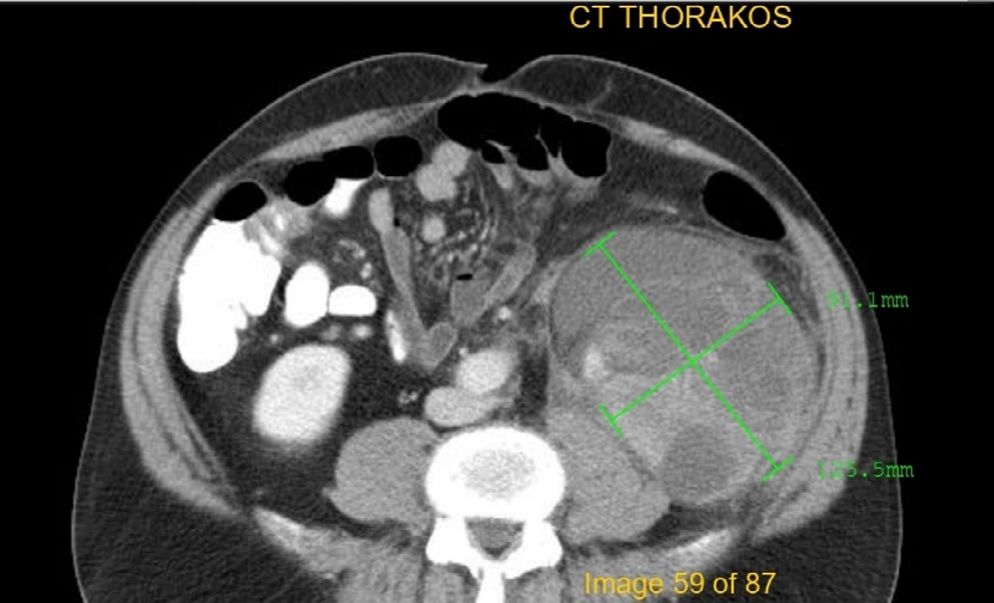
Sixty‐two‐year‐old man with recurrent retroperitoneal liposarcoma; CT scan shows a soft‐tissue mass in retroperitoneum. The longest diameter is 12.5 cm
